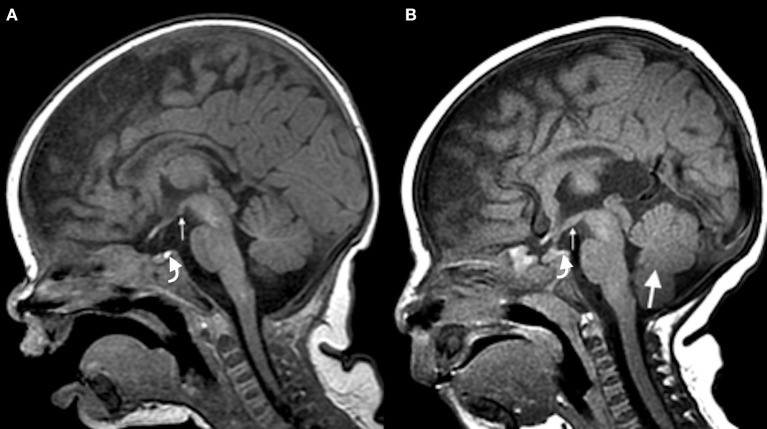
Sagittal T1-weighted brain MRI. (A) Normal MRI showing a homogeneous and marked signal hyperintensity pattern of the sellar region (curved arrow) in a 2 month-old infant and a normal hypothalamus (small arrow). (B) Patient's MRI showing a diminutive and barely discernible posterior pituitary hyperintensity (curved arrow), hypothalamic malformation (small arrow), and hypoplastic vermis (large arrow).
